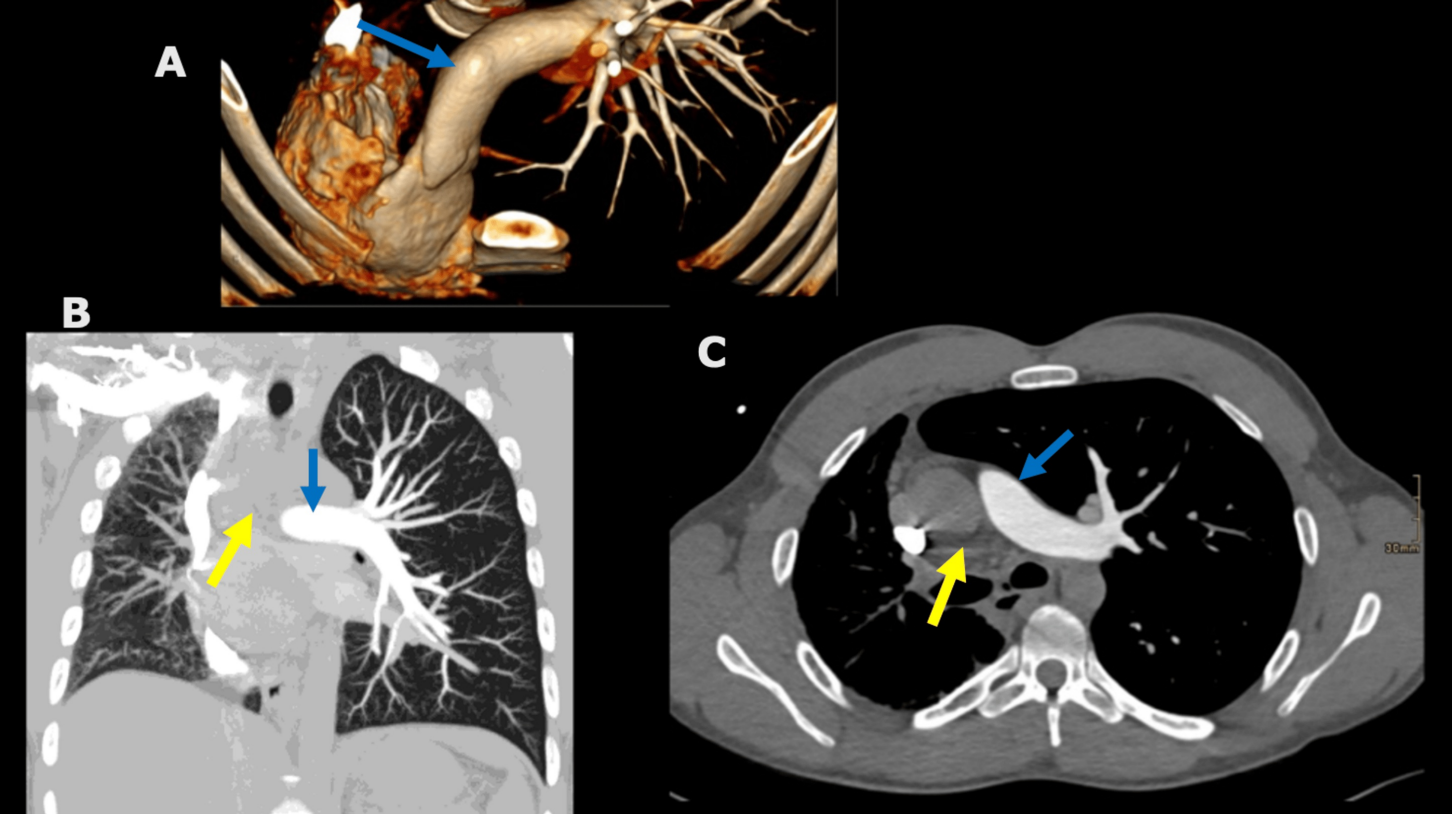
Absence of right pulmonary artery Axial 3-dimensional volume rendering (A), coronal (B), and axial (C) images of CT chest angiogram. Normal appearing left pulmonary artery (blue arrow) and missing right pulmonary artery (yellow arrow) are demonstrated.
